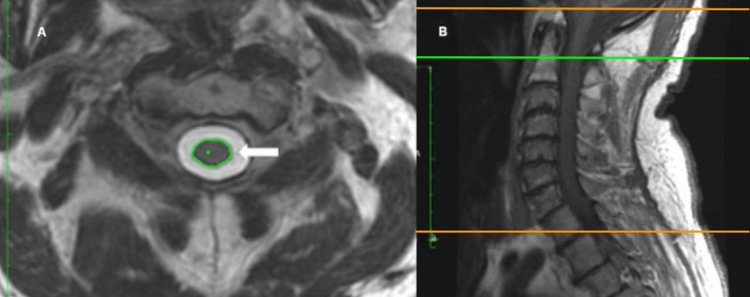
Spine MRI axial T2-weighted image at the level of mid-C2 vertebral body shown on the left. Automatic segmentation tool (arrow) was used to acquire cross-sectional areas of the spinal cord at mid-C2 to C7 vertebral levels (panel A). Midline sagittal T1-weighted image is shown on the right with reference lines (panel B) MRI: magnetic resonance imaging
